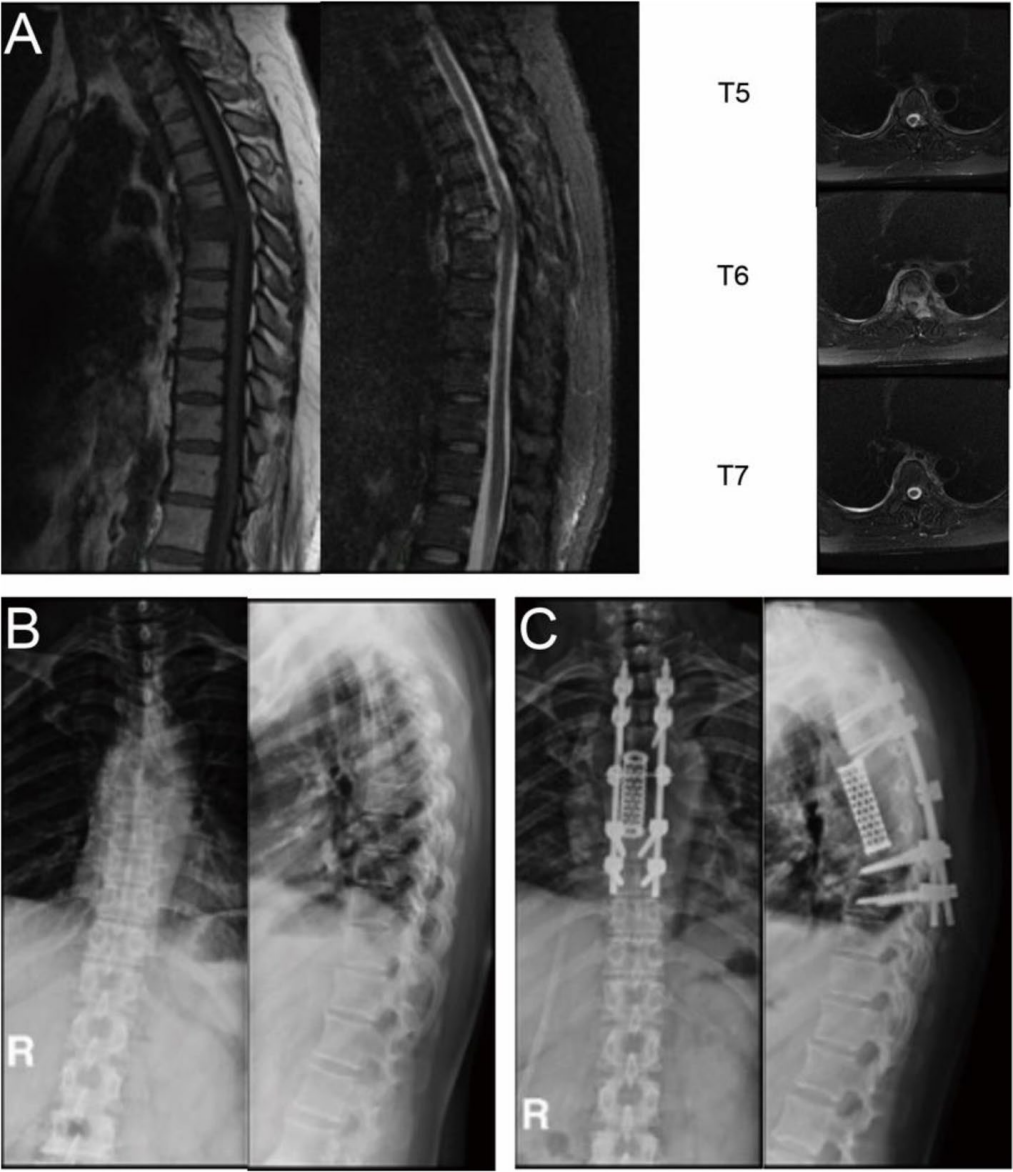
A 49-year-old woman with spine metastases of breast cancer. The patient underwent posterior marginal excision (en-bloc). The total blood loss was 4121 ml. A Preoperative sagittal thoracic vertebral MRI showed vertebral collapse at T6. Preoperative transversal MRI showed that the tumor involved the upper and lower adjacent vertebral bodies (T5 and T7); B Preoperative anteroposterior thoracic vertebral radiography; C Postoperative lateral anteroposterior thoracic vertebral radiography
